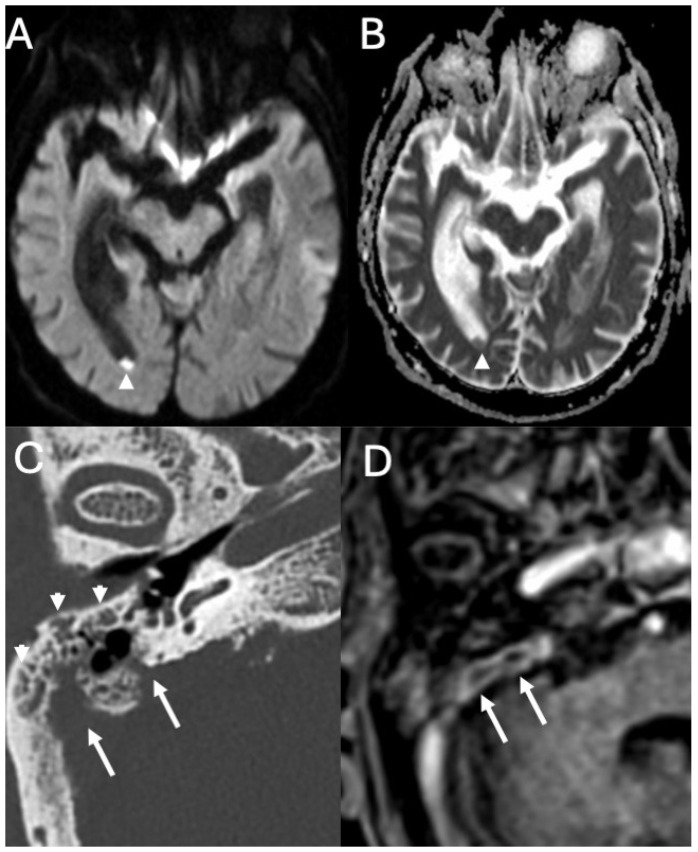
Meningitis due to *Streptococcus pneumoniae* in an 82-year-old. Axial DWI (A) with relative ADC map (B), CT (C), and enhanced T1 (D) depict intraventricular purulent material (arrowheads in (A,B)). Note also right acute mastoiditis (arrowheads in (C)) with extensive bony erosion (arrows in (C)) and intense contrast-enhancement (arrows in (D)).
